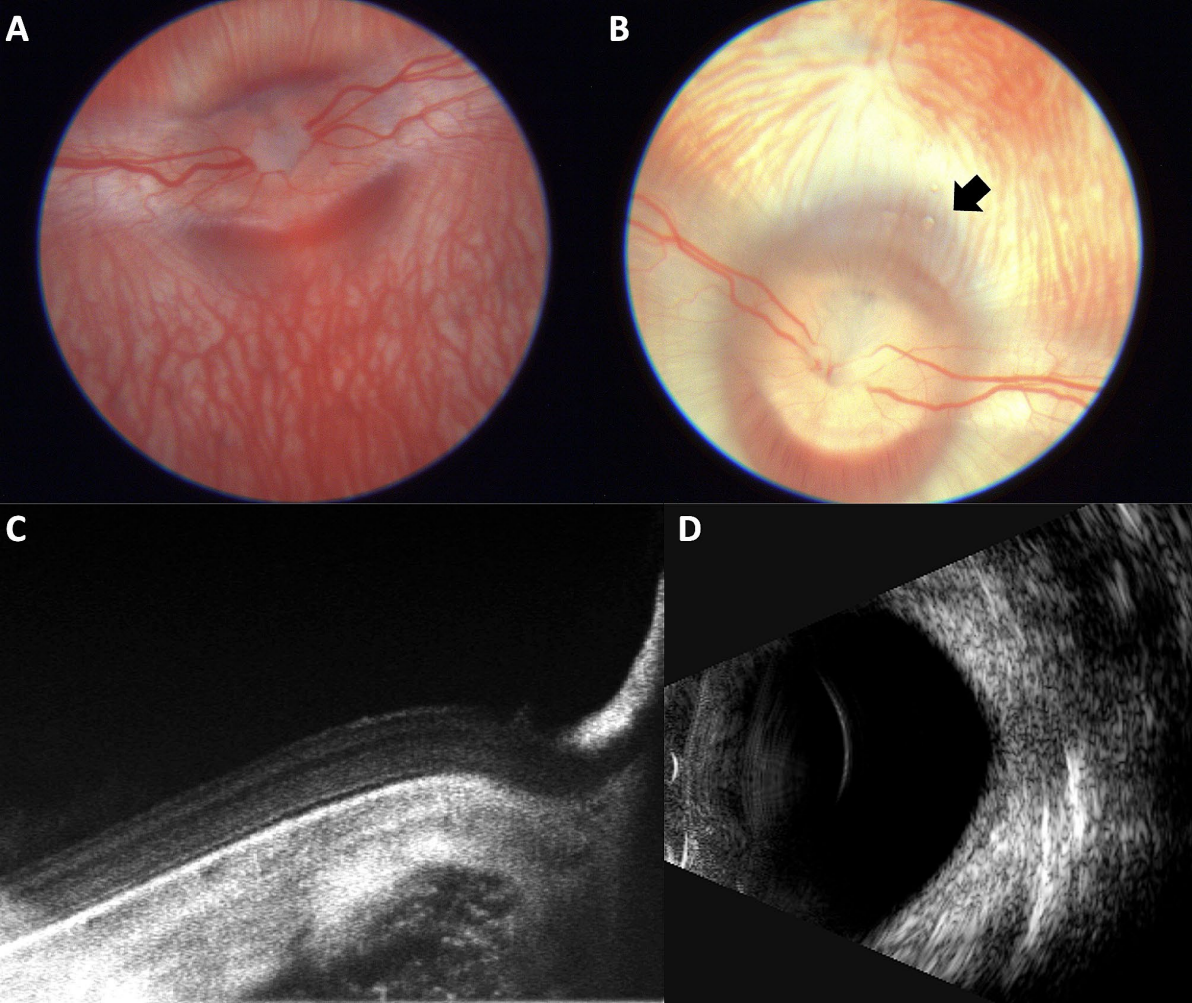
Retinal images following Nd:YAG laser vitreolysis. A. Color photo of the retina before Nd:YAG laser vitreolysis; B. Color photo of the retina after YAG laser vitreolysis, gas bubbles (black arrow) were noted above the optic disc; C. Retinal OCT of the treated eye three months after Nd:YAG laser vitreolysis, clear retinal layers were noted without any damage; D. Ultrasound B scan of the treated eye three months after Nd:YAG laser vitreolysis, no unmistakable echo of the vitreous cavity was found
